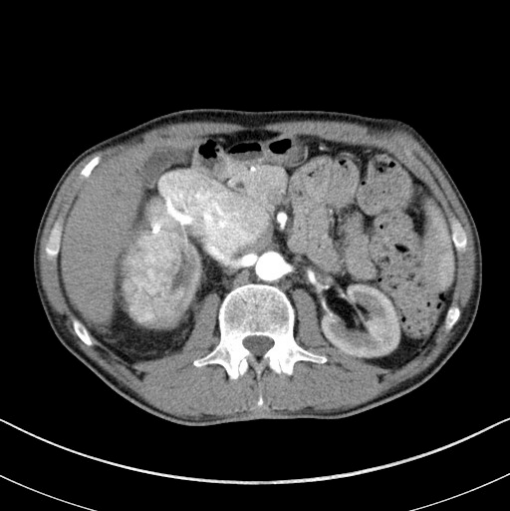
CT scan showing involvement of infradiaphragmatic juxtahepatic part of inferior vena cava.
